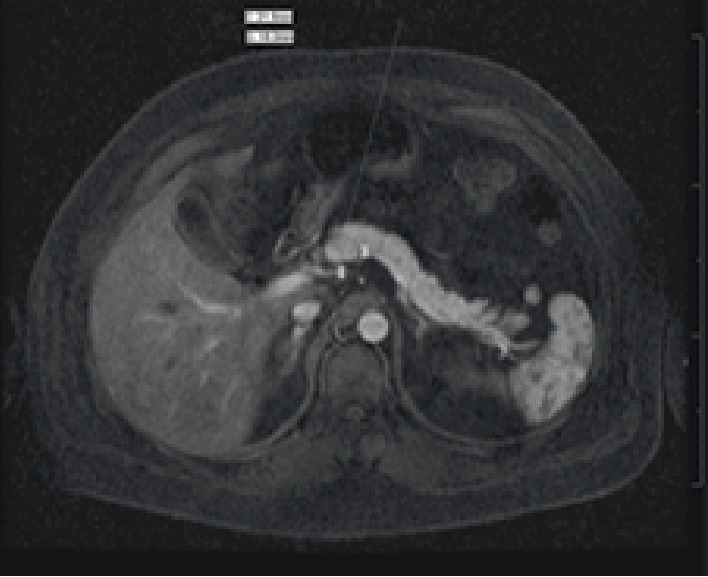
Magnetic resonance imaging T1 early arterial phase with arrow indicating mass.
